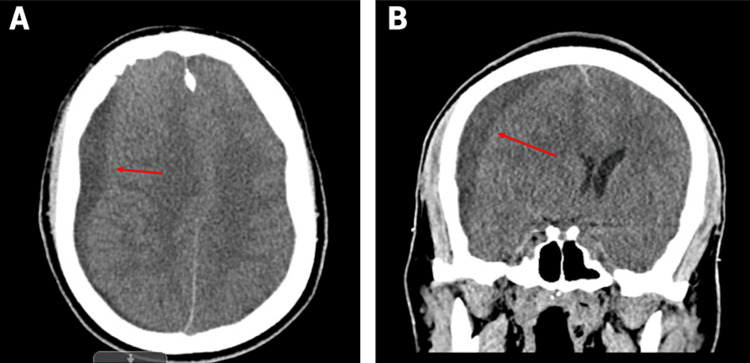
Initial CT imaging showing subdural empyema: (A) transverse view and (B) coronal view
